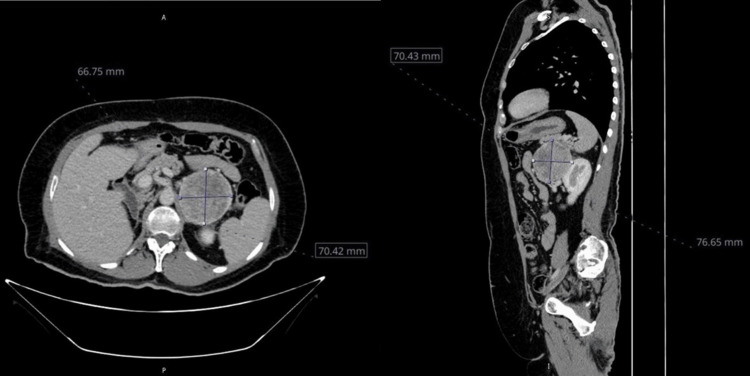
Progressive enlargement of the left adrenal lesion on contrast-enhanced CT Contrast-enhanced CT scan of the left adrenal gland (February 2025) showing further enlargement of the lesion to 70 × 76 × 70 mm. The lesion demonstrates internal necrosis and post-contrast enhancement (69 HU in the venous phase, 62 HU in the delayed phase). No additional metastatic lesions were identified. Left panel: coronal view. Right panel: sagittal view.
